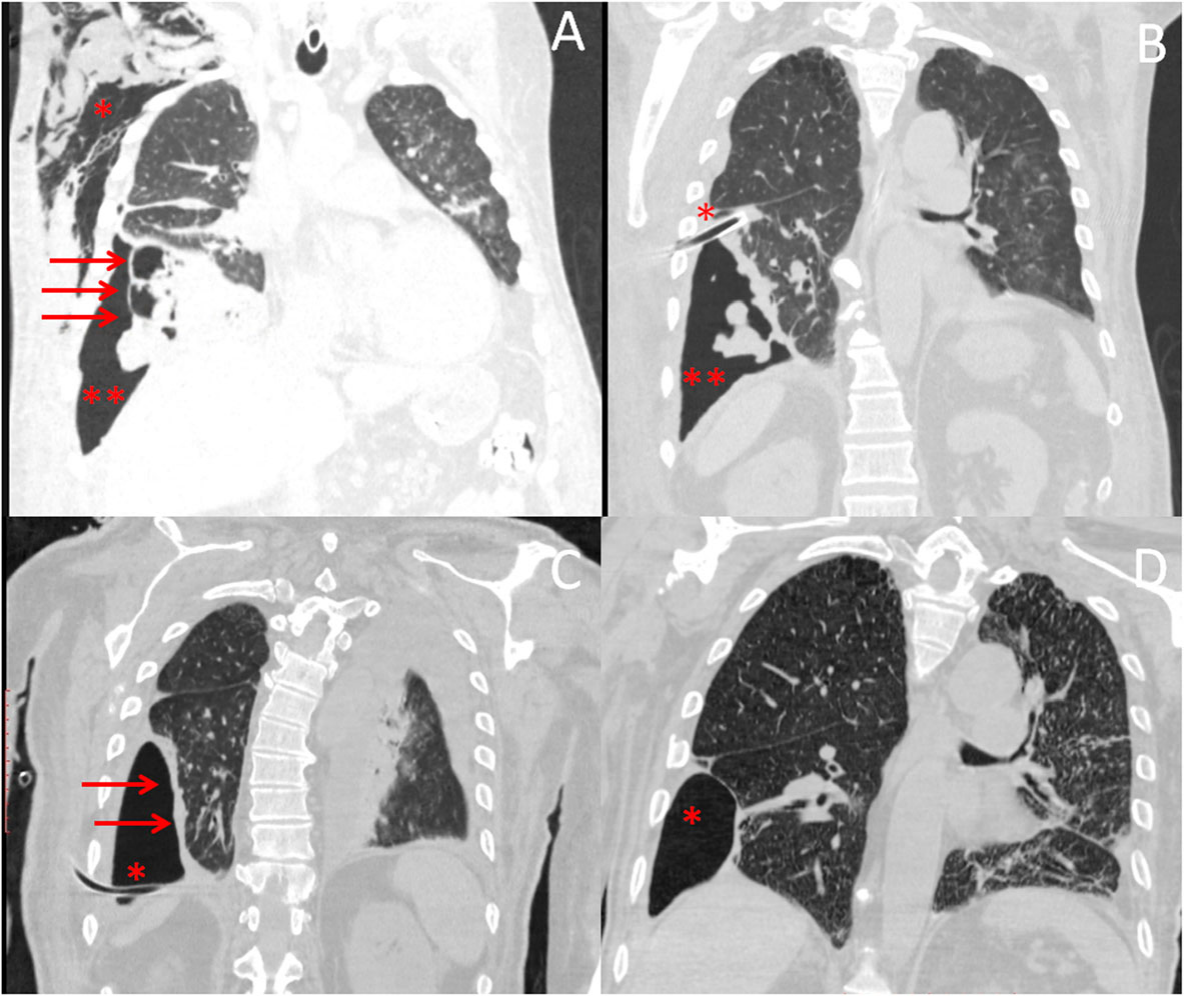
Chest computed tomography scan showed the presence of subcutaneous emphysema (*), pneumothorax (), and necrotizing pneumonia with empyema (arrows) (Part a). After chest drainage placement (*), computed tomography scan showed the persistence of loculated pneumothorax () (Part b). Despite the insertion of chest tube (*), right lower lobe did not expand as it was trapped by pleural adhesions (arrows) (Part c). Following closure of alveolar pleura fistula, chest computed tomography showed no progression of loculated pneumothorax (*) (Part d)
